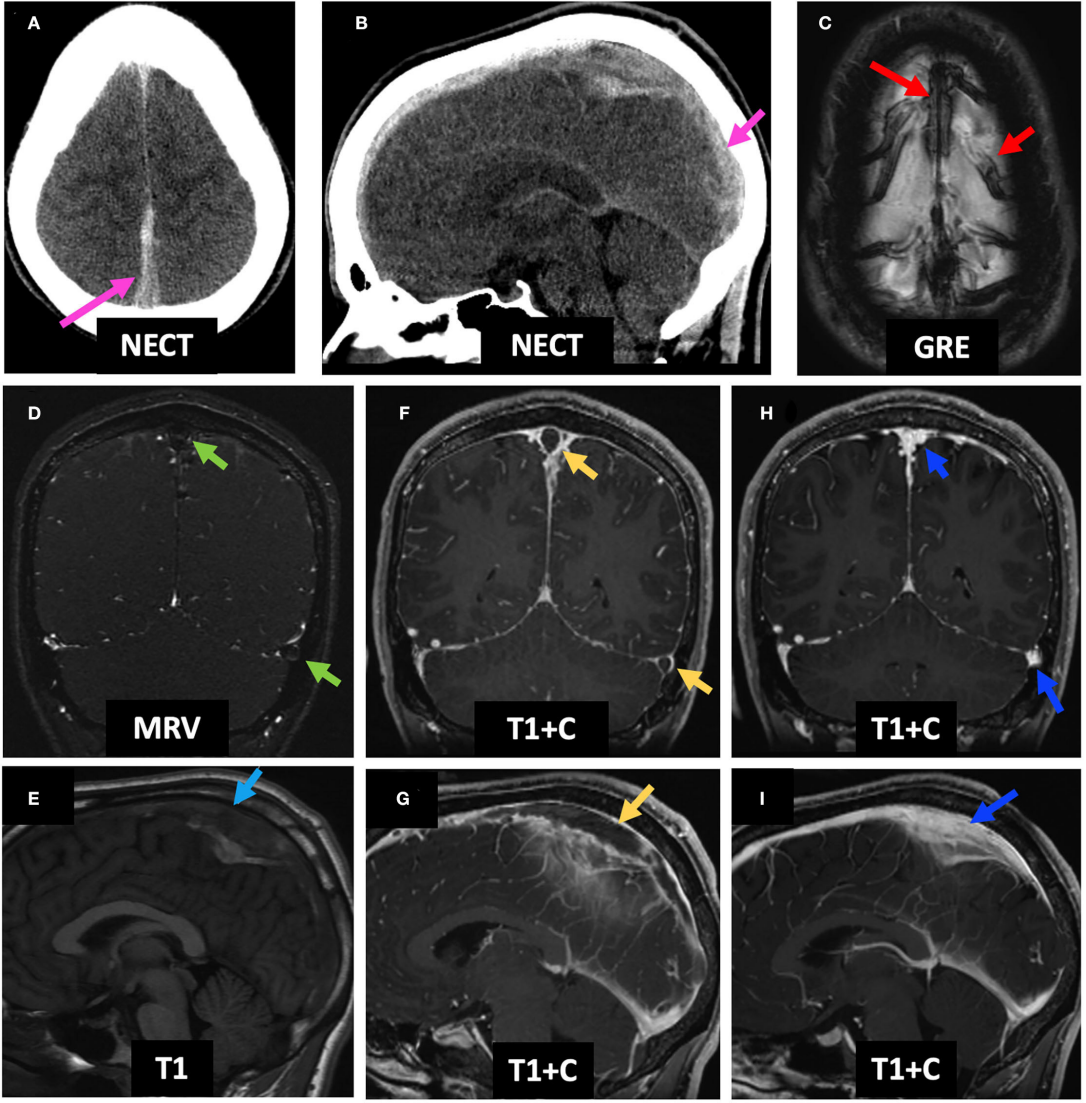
Case 1: An 18-year-old male who underwent a lumbar puncture 1 week prior to presentation was found to have worsening headache, dysarthria, and right hemiparesis with papilledema on exam. Initial NECT demonstrates hyperdensity of the SSS (A,B, pink arrows). C-MRI demonstrates GRE+ in the SSS and adjacent cortical veins (C, red arrows), lack of enhancement on MRV (D, green arrows), an expanded and T1 isointense SSS (E, light blue arrow), and empty delta sign in the SSS and left TS (F,G, yellow arrows). He received anticoagulation for 6 months. Follow-up C-MRI 4 months post-initial presentation demonstrates resolution of the GRE hypointensity and filling defects within the SSS and left TS, consistent with recanalization (H,I). LP, lumbar puncture; NECT, non-contrast-enhanced CT; SSS, superior sagittal sinus; C-MRI, contrast-enhanced MRI; MRV, MR venography; TS, transverse sinus.
